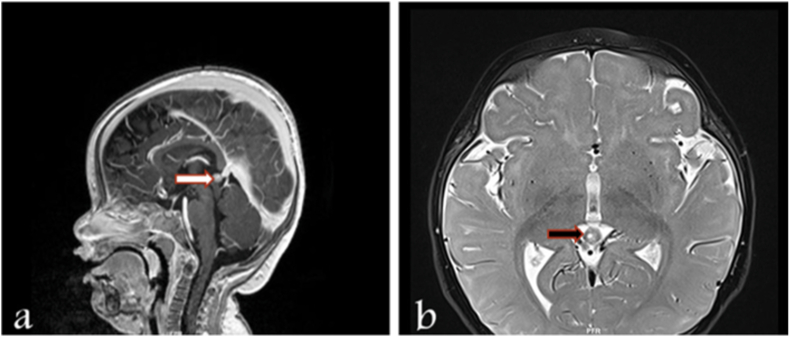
MRI brain findings of case 2 demonstrating pineal involvement (a) sagittal plane (b) axial plane. The pineal gland was relatively prominent (arrow) with heterogeneous appearance and enhancement. No sellar or suprasellar mass was noted.
